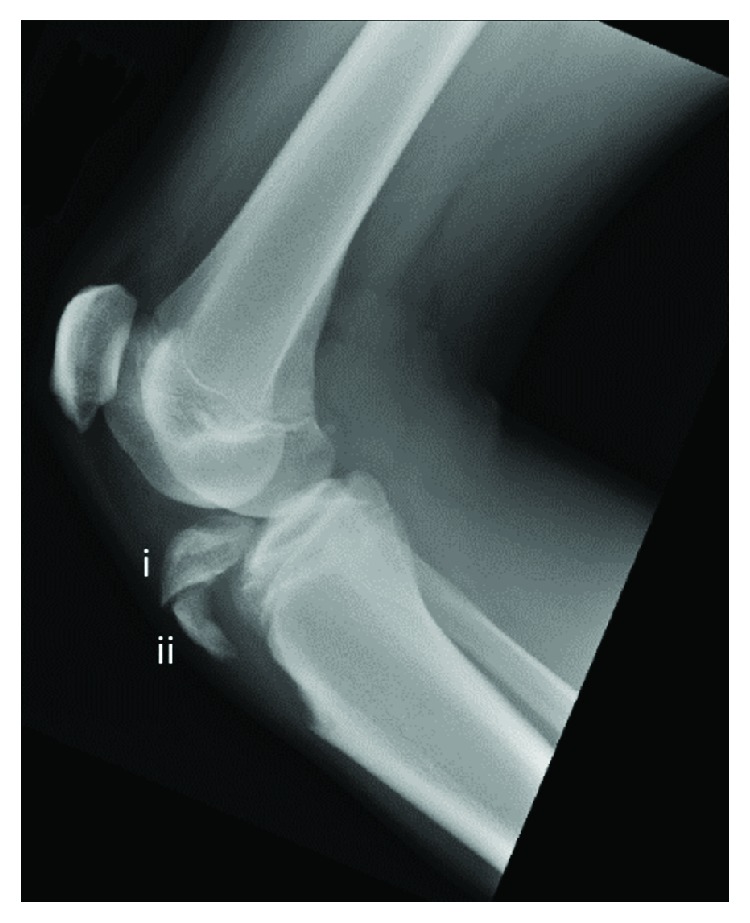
Preoperative lateral X-ray with knee at 30° flexion demonstrated tibial tubercle avulsion fracture extending into the joint space with two primary fragments: (i) anterior tibial plateau and (ii) tibial tubercle.
